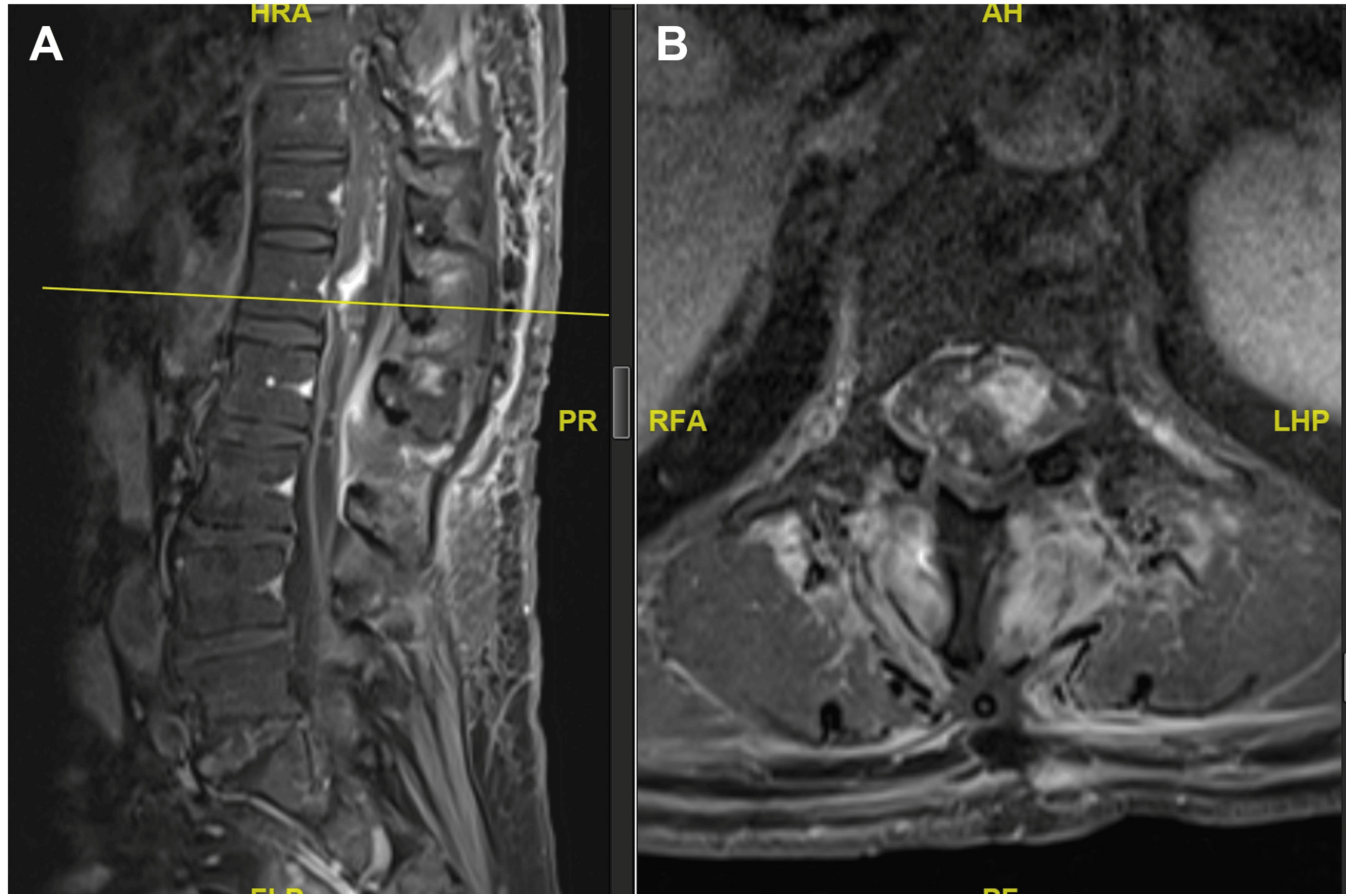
Postoperative day one: MRI of the T1-weighted imaging showing a residual tumor at the T11-12 level
